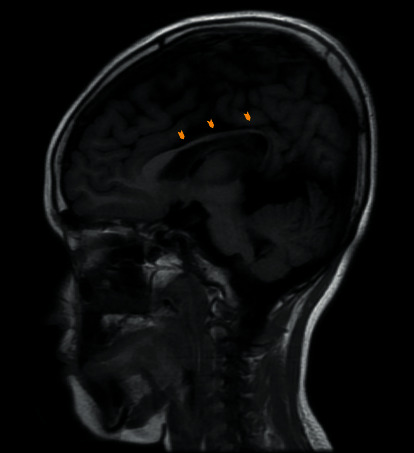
Sagittal slice of T1-weighted image showing dysgenesis of the corpus callosum (arrowheads).
